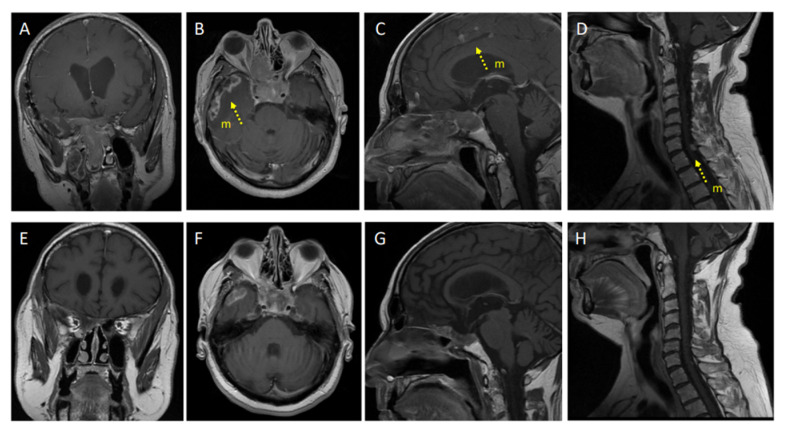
Radiological response to pembrolizumab: Shown are representative MRI obtained before (A–D) and after 1 year of treatment (E–H) in the patient affected by a silent metastatic Pit1-positive PitNET. Coronal (A,E) and axial (B,F) T1-weighted sequences post-gadolinium, show a regrowth of the sellar mass inferiorly with nasal obstruction (A), with anterior spread to the right retrobulbar optic area (B), and tumor shrinkage (>70%) after pembrolizumab with the persistence of minimal tissue of uncertain significance at the primary site (E,F). Sagittal T1-weighted sequence post-gadolinium (C,G) showing brain dissemination with the appearance of multiple, small and asymptomatic multiple metastases (m, yellow arrow)—the necrotic aspect of a metastatic site which previously received stereotactic radiotherapy along with metronomic temozolomide can also be recognized in (C), and their subsequent disappearance after pembrolizumab. (G) Spinal sagittal T1-weighted sequence post-gadolinium (D,H) showing the progression with the main spinal metastasis at C6–D1 (D) (yellow arrow) which nearly disappeared after treatment (H).
